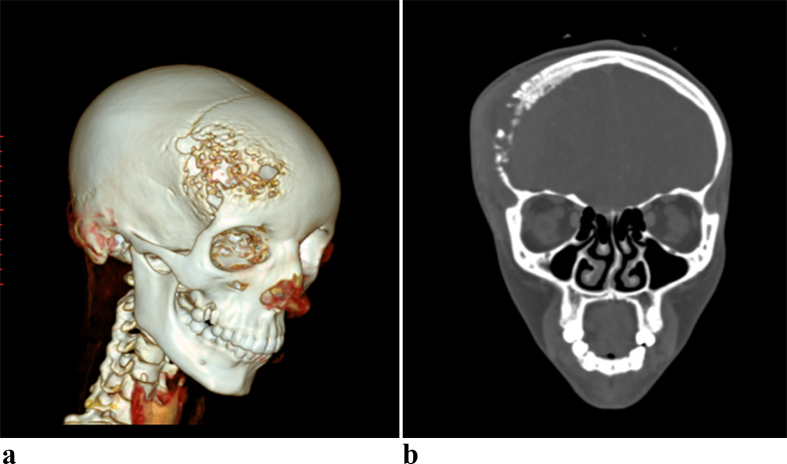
a. 3D reconstruct of Computed tomography showing multiple bony defect of the right frontal bone b. Bone window of Computed tomography showing right frontal bone destruction with sub galeal swelling.
